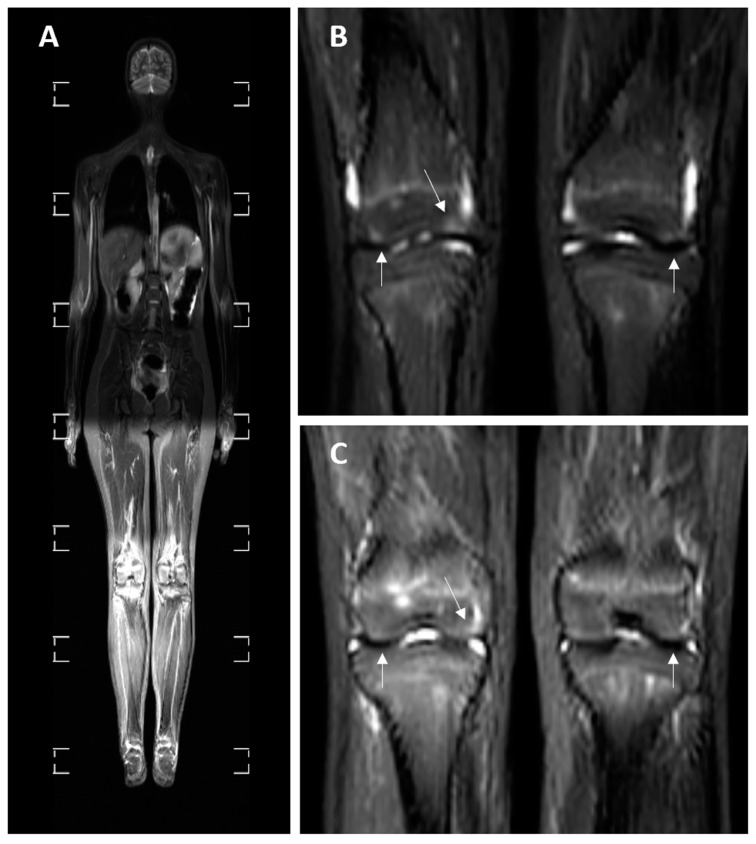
Whole-body MRI in the STIR sequence of a 14-year-old female patient with JIA and a history of knee joint pain. (A) A whole-body MRI scan. (B,C) Two consecutive cross-sections of the knee joints. A small amount of knee joint effusion and the signal elevation in the right femur epiphysis are notable on the images, pointed by white arrows (B,C).
